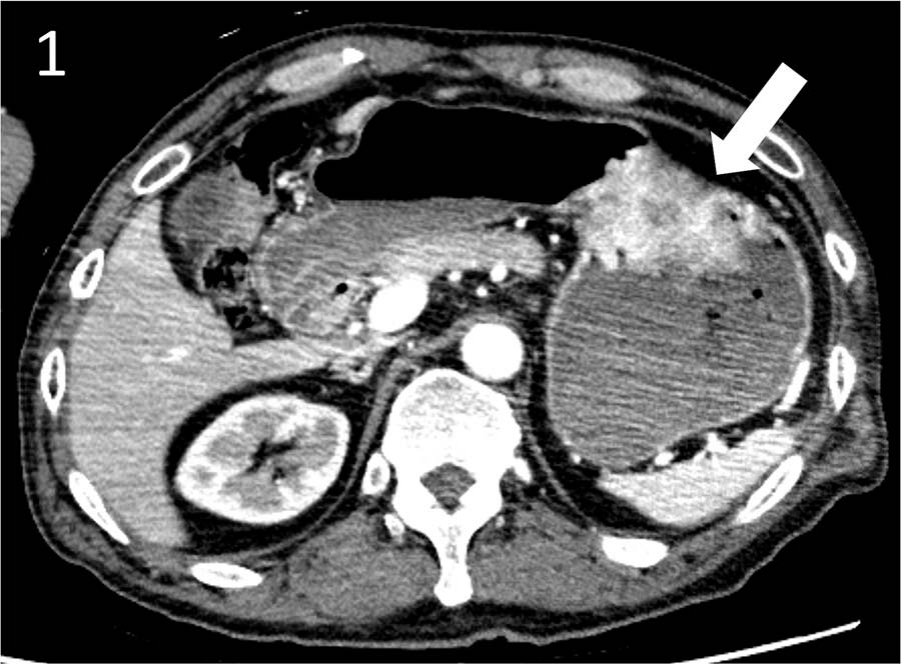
Contrast-enhanced computed tomography image showing a gastric hyper vascular mass on the lesser curvature (arrow).
